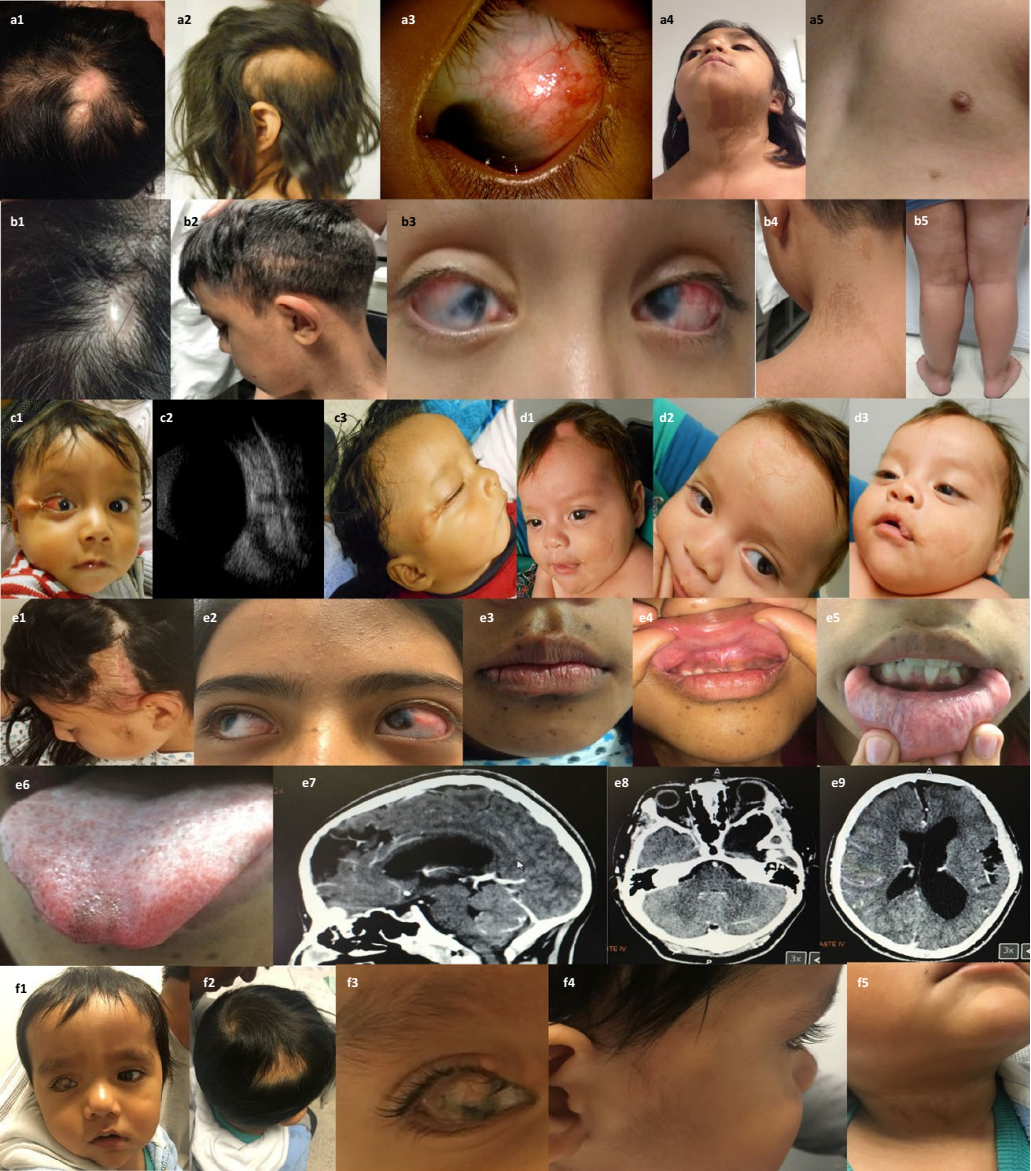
Clinical characteristics of patients with oculocutaneous mosaic RASopathies. (a) OES patient #1. (a1) Two aplasia cutis verticis lesions; (a2) Left parietal alopecia; (a3) Epibulbar dermoid on the left eye; (a4) Hyperpigmentation on left mandibular region and left side of the neck; (a5) Polythelia. (b) OES patient #2. (b1) Aplasia cutis verticis; (b2) Temporal‐parietal alopecia; (b3) Bilateral epibulbar dermoid; (b4) Cutaneous hyperpigmentation on the posterior neck; (b5) Lymphedema of left limb. (c) ECCL patient #3. (c1) Nevus psiloliparus on right zygomatic region; (c2) Right optic nerve coloboma; (c3) Alopecia frontal, parietal, and nevus psiloliparus on the temporal‐parietal regions. (d) SFMS patient #4. (d1–3) Nevus sebaceous on the left parietal‐frontal‐maxilar, and upper lip regions. (e) SFMS patient #5. (e1) Nevus sebaceous on the left temporal‐parietal regions; (e2) Epibulbar dermoid in left eye; (e3) Hyperpigmentation around the mouth, and multiple hyperpigmented nevi inside the cutaneous pigmentation lesion are evident; (e4–6) Hyperpigmentation of upper and lower gingival mucosa as well as in the tip of the tongue. (e7) Cranial computed tomography evidenced a left temporal arachnoid cyst, (e8) a left eye retinal coloboma, and (e9) hemimegalencephaly; (f) ECCL patient #6. (f1) Facial appearance. Brachycephaly was noted; (f2) Alopecia in right parietal region; (f3) Right eye: epibulbar dermoid and small nodular tag in the upper lid; (f4) Skin hypoplasia in the right zygomatic and preauricular regions; (f5) Skin linear hyperpigmentation in the neck
